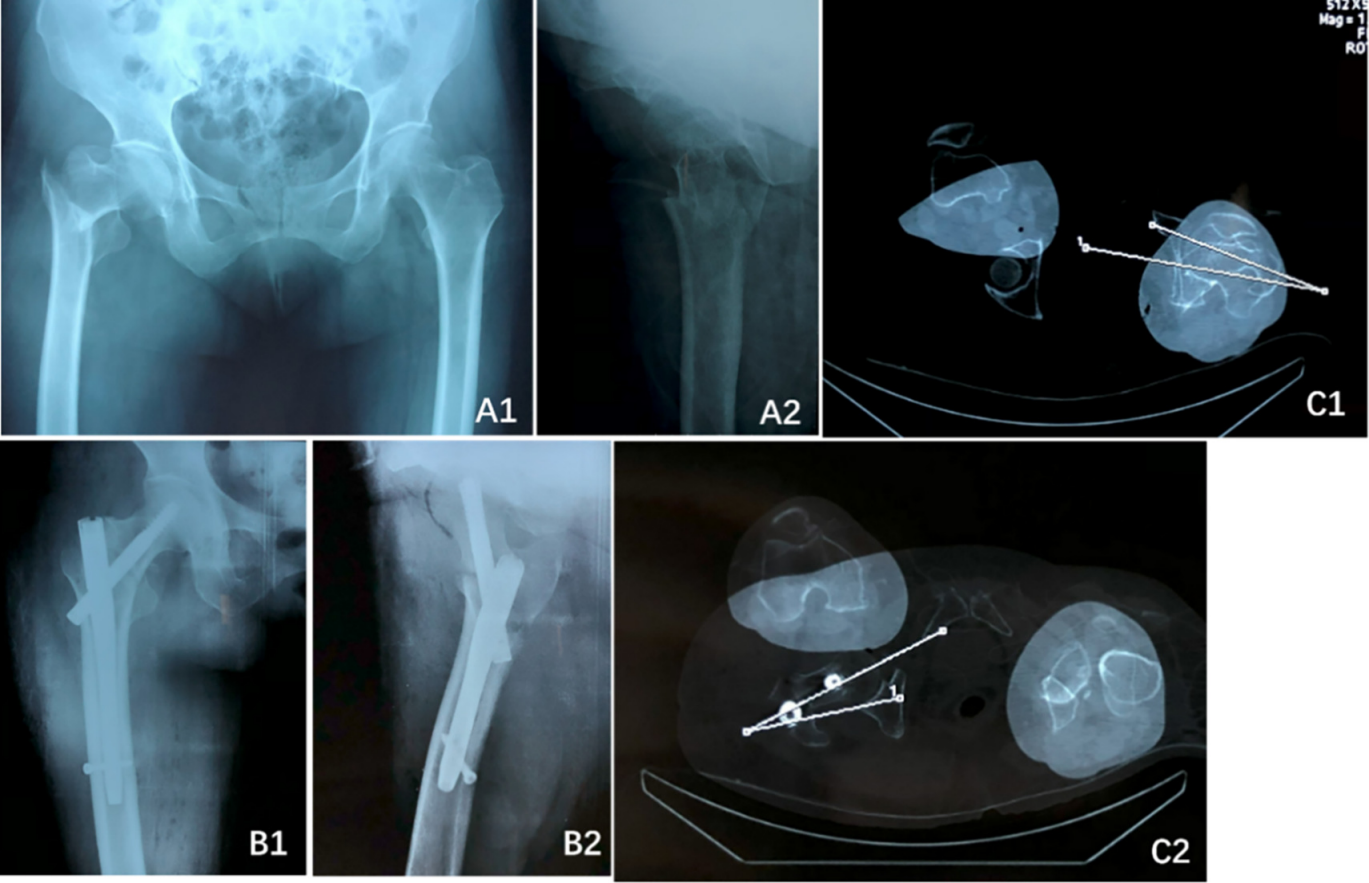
A 75-year-old female patient with a right femoral intertrochanteric fracture (AO/OTA type 31-A2.2). (A1) Preoperative anteroposterior (AP) radiograph; (A2) Preoperative lateral radiograph; (B1,B2) Postoperative AP and lateral radiographs 2 days after surgery, demonstrating excellent fracture reduction and fixation; (C1) Measurement of anteversion in the healthy contralateral limb; (C2) Measurement of anteversion in the affected limb following internal fixation.
